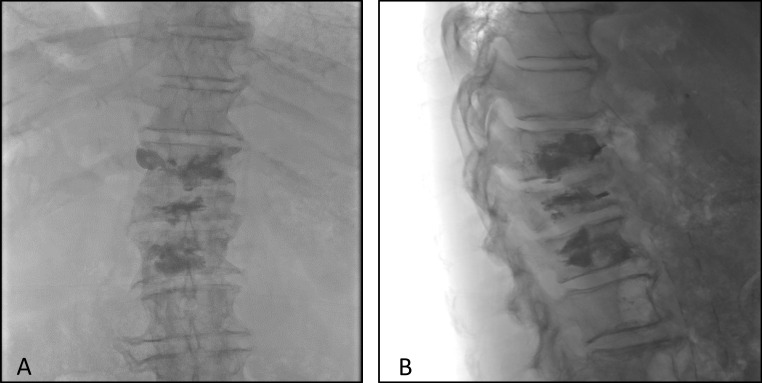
AP (A) and lateral (B) radiographic views post radiofrequency ablation and vertebral augmentation
